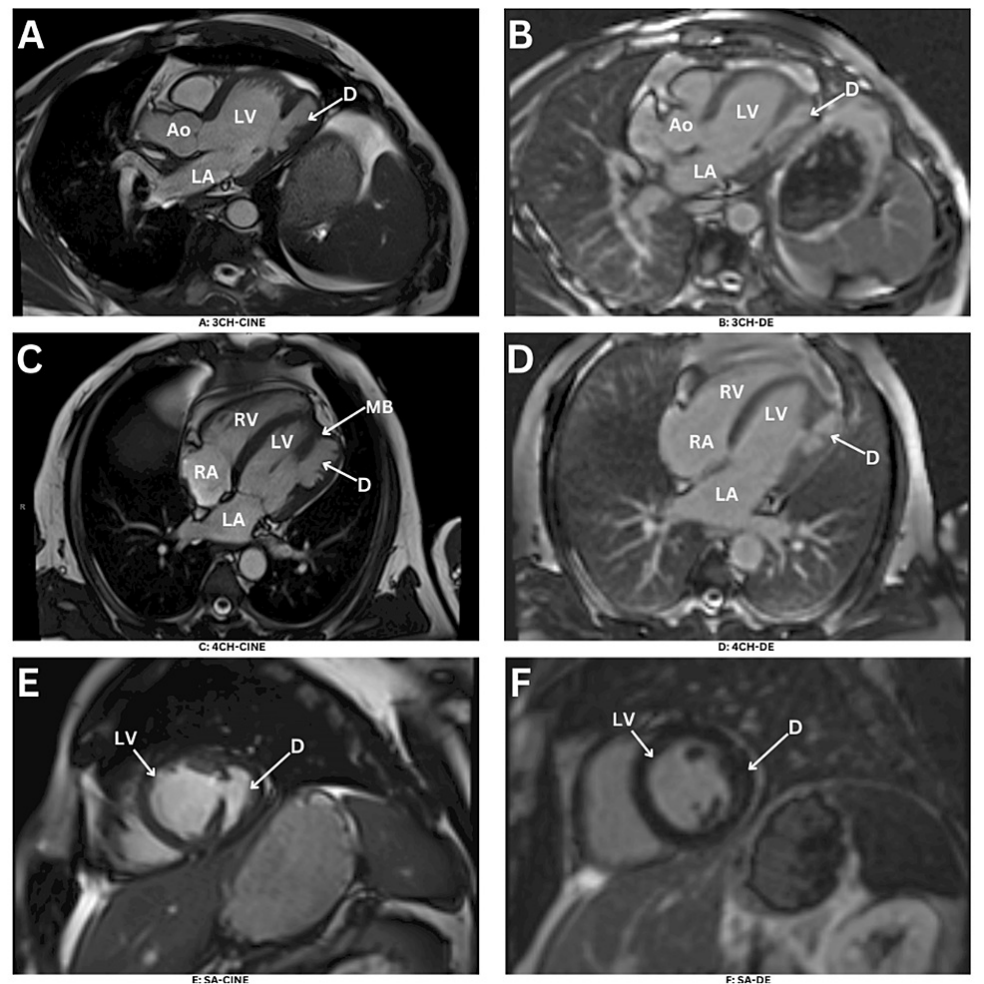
Cardiac magnetic resonance imaging Cardiac MRI demonstrated the left ventricle diverticulum myocardial wall with noticeable wall thinning measuring 2-3 mm in the lateral area in the four chambers long axis SSFP view (C), and D (DE). Three chambers view SSFP sine (A) and (B) of DE. Short axis SSFP (E) and F (DE). D, diverticulum; LA, left atrium; LV, left ventricle; MB, muscle band; RA, right atrium; RV, right ventricle. DE (delayed contrast enhancement). SSFP: steady-state free precession sequence.
